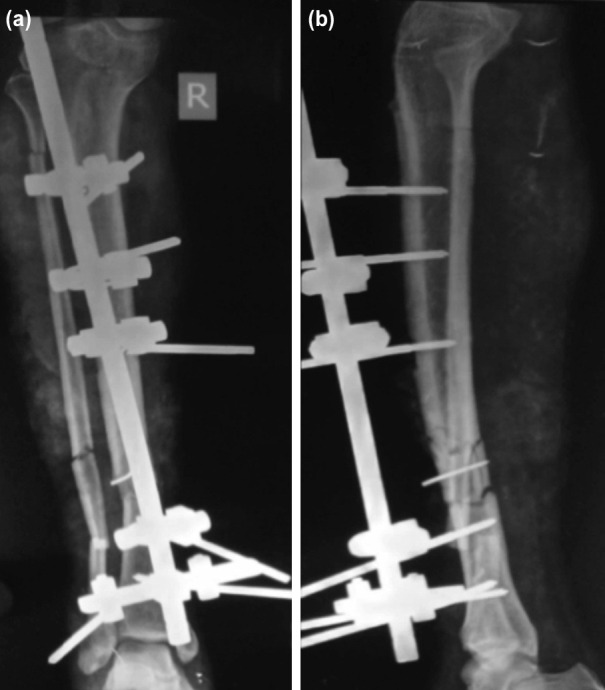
(a) Anterior posterior and (b) lateral plain radiograph showing tibia fracture treated with debridement and external fixation.
